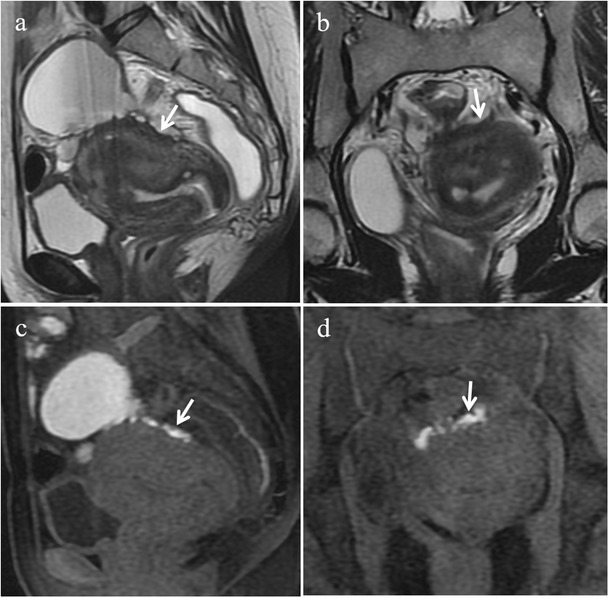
Endometriosis of the uterine serosa in a 46-year-old woman, who underwent a previous surgical intervention for endometrioma of the right ovary. (a) Sagittal and (b) oblique axial T2-weighted images show endometriotic implants involving the uterine serosa. The lesions demonstrate indistinct margins and low signal intensity (white arrows). On (c) sagittal and (d) coronal fat-suppressed T1-weighted images the lesions display high signal intensity (white arrows)
